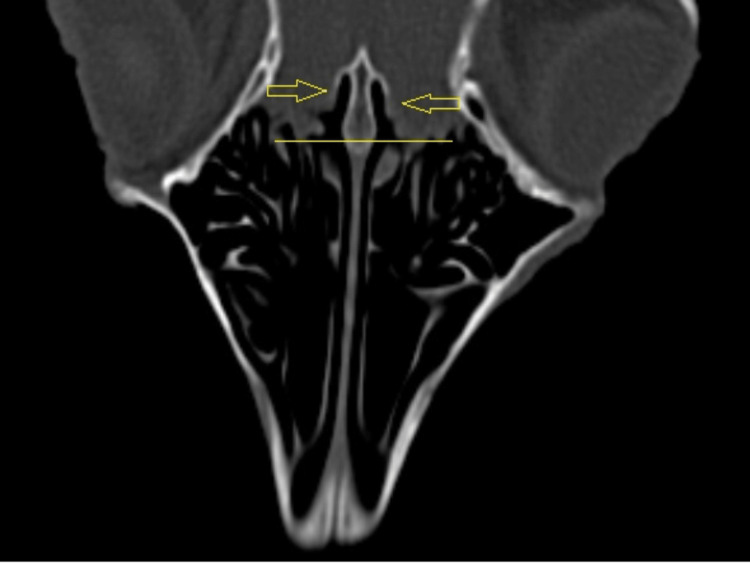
Coronal CT scan: level of the ethmoidal labyrinth The ethmoidal roof corresponds to Keros type 3 from human anatomy.
